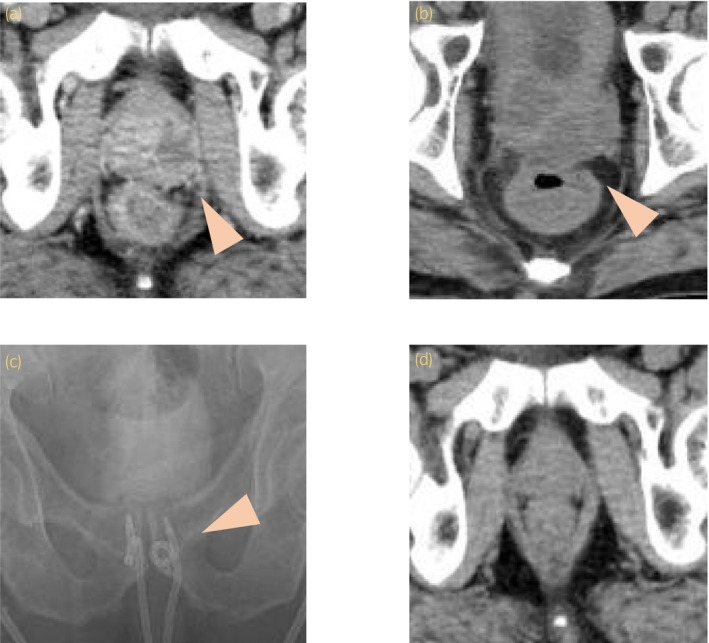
(a) PA (left lobe: 25 × 21 mm) was in contact with the rectum. (b) On CT during the exacerbation of inflammation, the abscess spread to both lobes of the prostate and was in contact with the rectal wall. (c) Two pigtail catheters were placed in the prostate. (d) CT of 6 months after the catheter removal shows improvement of the PA.
